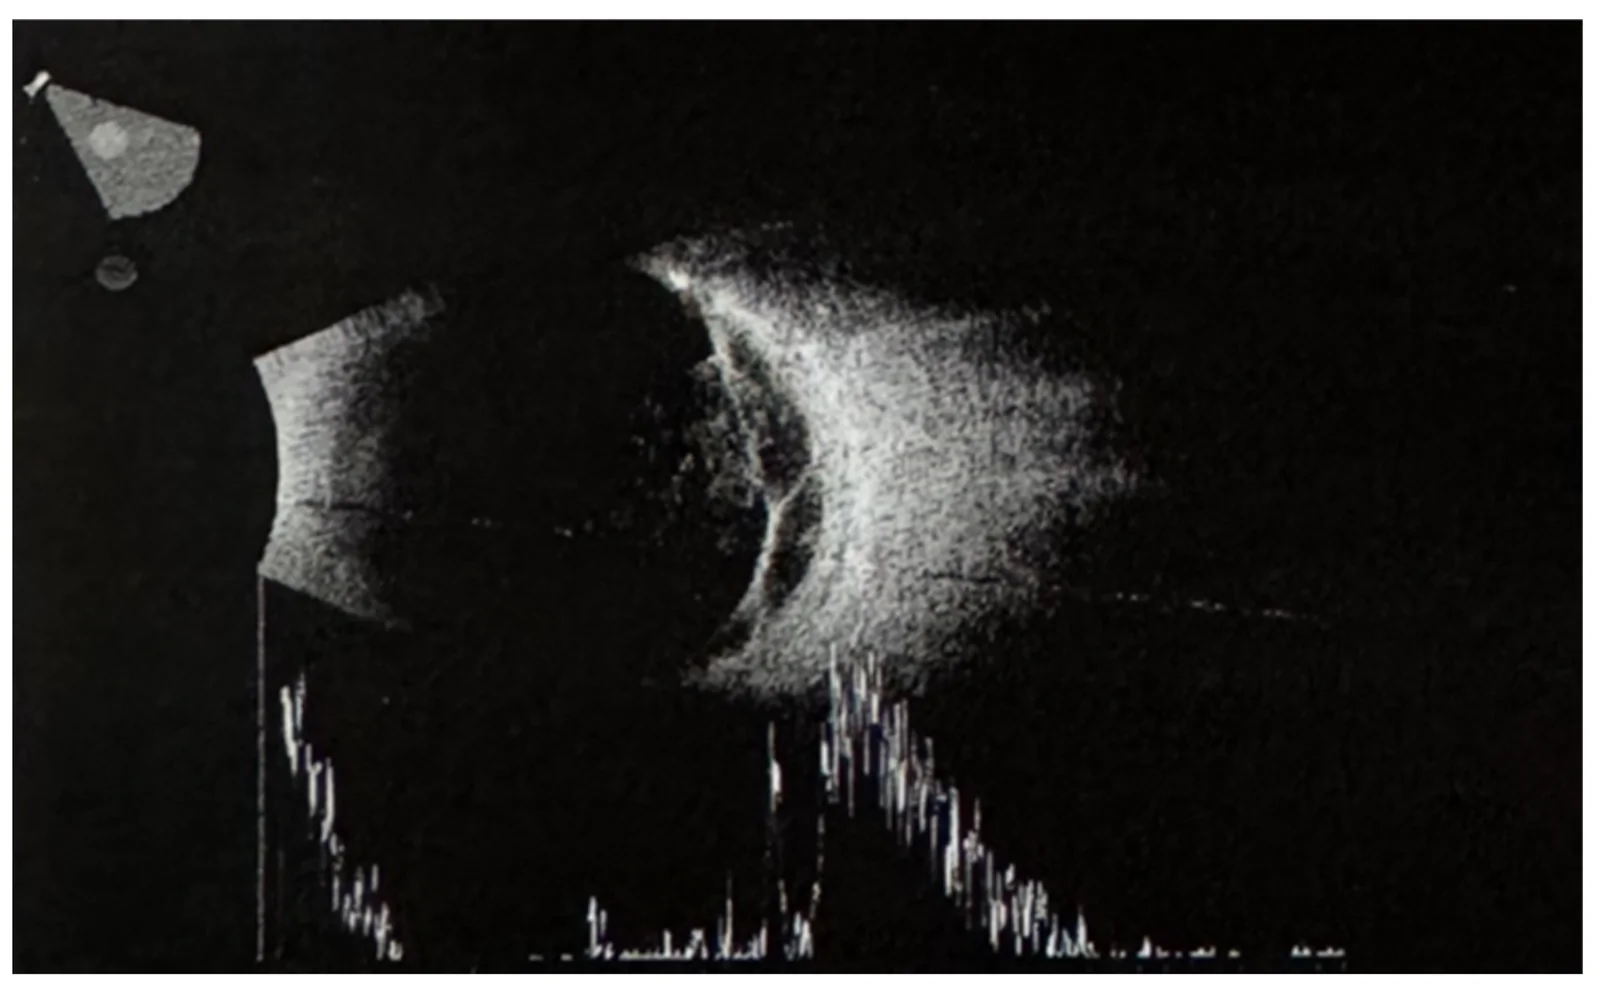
Ultrasound scan of the right eye of patient 3.
